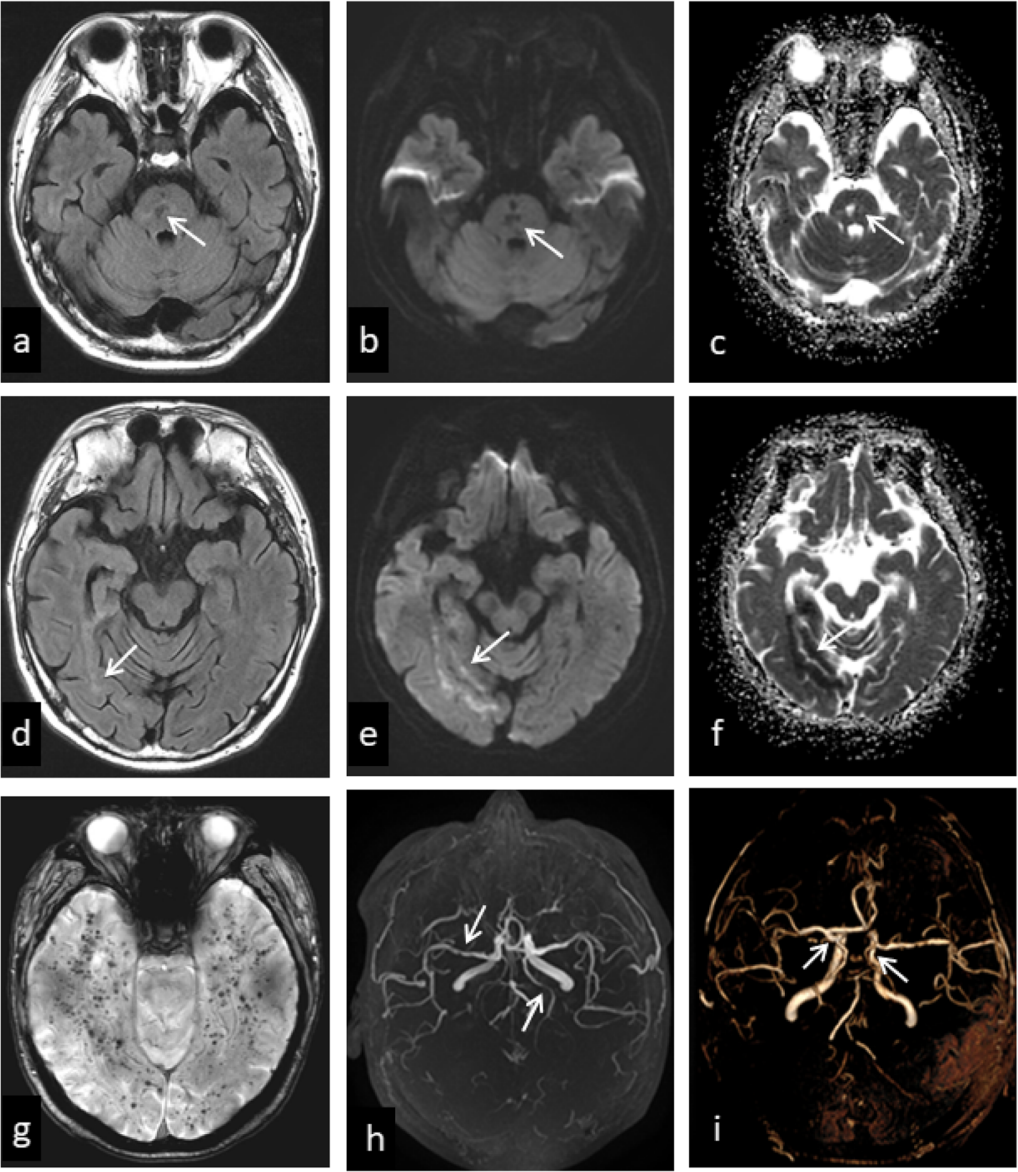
Brain magnetic resonance imaging (MRI). a-c: Infarction in brainstem with hypointense signal in fluid attenuated inversion recovery (FLAIR), hypointense in diffusion weighted imaging (DWI) and hyperintense signal in apparent diffusion coefficient (ADC). d-f: Acute ischemic stroke in temporal lobe and occipital lobe with relatively low signals in FLAIR, hyperintense signal in DWI and hypointense signal in ADC. g-i: CMBs in the susceptibility weighted imaging (SWI) sequence. Multiple localized stenosis of the right middle cerebral artery and the left posterior cerebral artery in MRA. The bilateral posterior cerebral artery originated from the bilateral internal carotid artery in MRA 3D reconstruction
